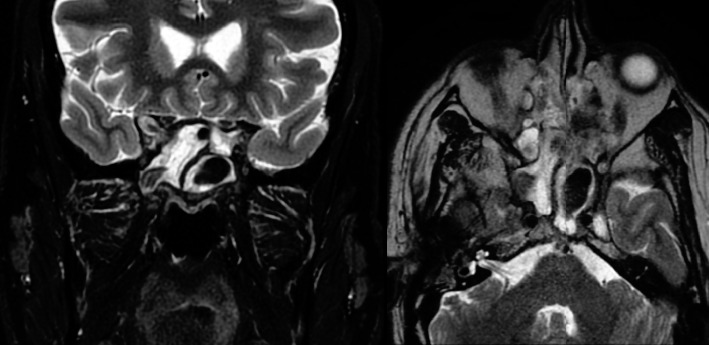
Coronal (left) and axial (right) *T*2 magnetic resonance imaging showed an abnormality from the lateral aspect of the right sphenoid sinus extending along the right superior orbital fissure.
